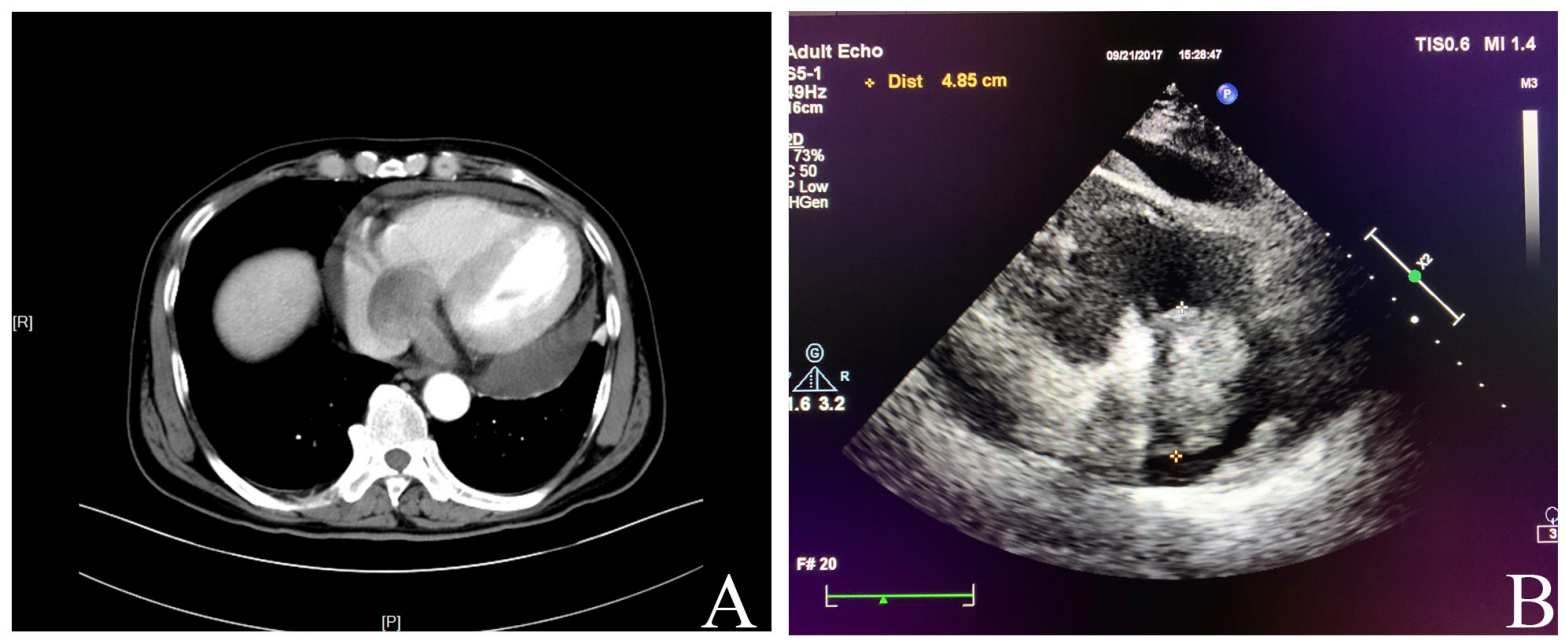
(A) Computed tomography showed an occupying lesion involving the right atrium and the right ventricle. (B) Echocardiography revealed a mass in the right atrium with moderate pericardial effusion.
